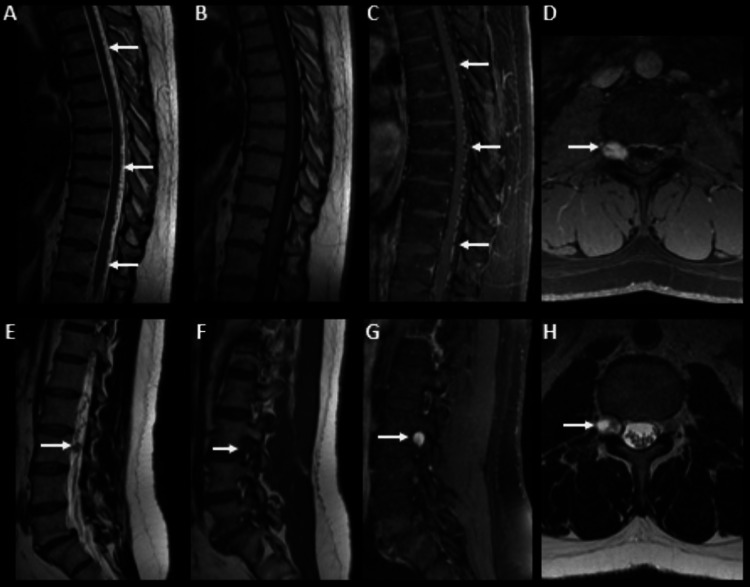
Magnetic resonance imaging of the lumbar spine (sagittal and axial views). Sagittal T2WI (A) of the thoracic spine shows multiple serpiginous flow voids (arrows), located in the dorsal aspect of the spinal cord, difficult to identify on sagittal T1WI (B), revealing intensely gadolinium-enhancement on sagittal T1WI fat-saturated (C, arrows). Sagittal T2WI of the lumbar spine (E) reveals caudal extension of the lesions, with a nodular lesion at L3 level (arrow) that extended to the right foraminal foramen, showing hypointense signal on sagittal T1WI (F), intense homogeneous enhancement after gadolinium (on axial (D) and sagittal (G) T1WI fat-saturated), and heterogeneous signal on axial T2WI (H, arrow).
